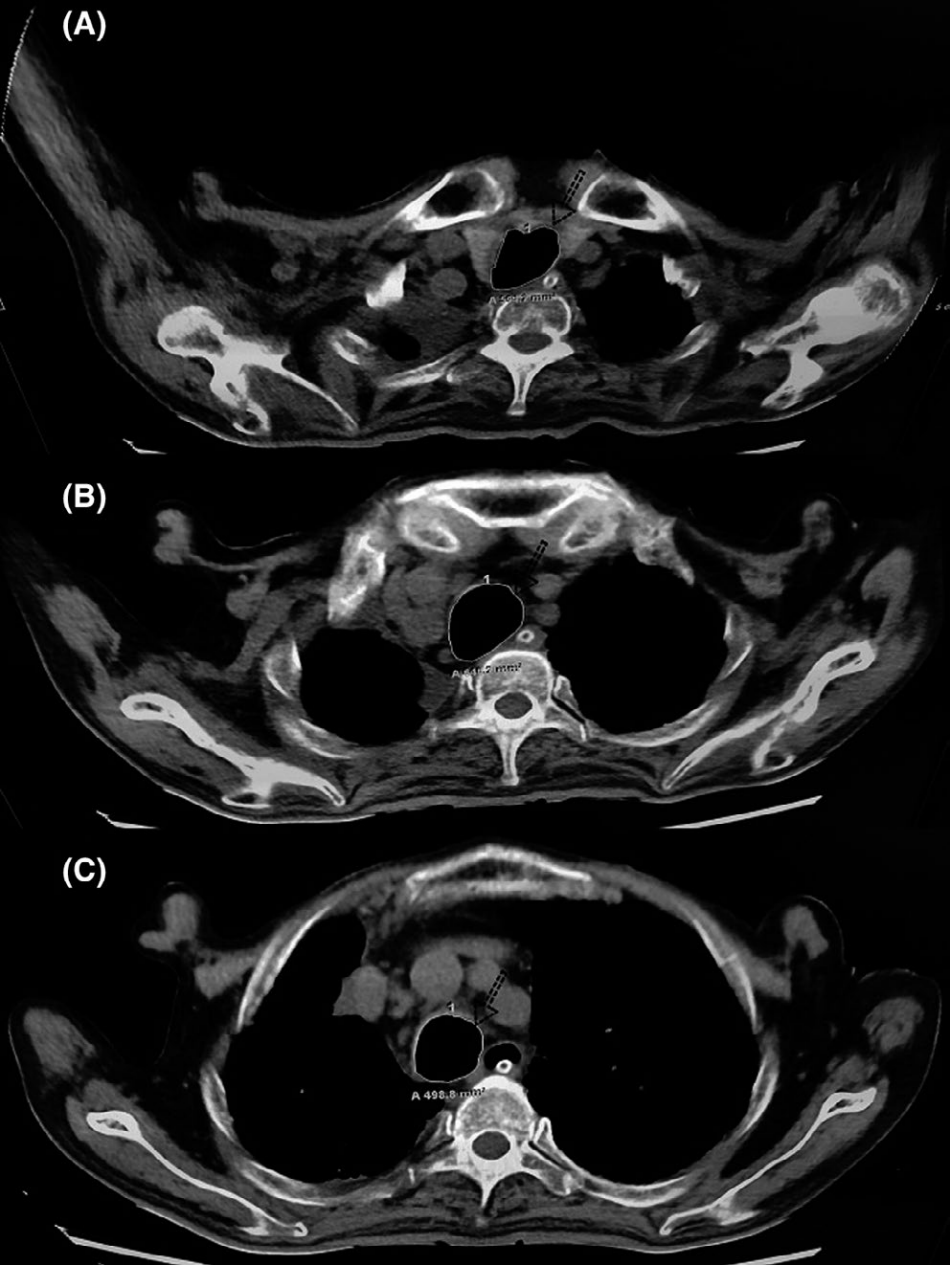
Computed tomography images used for measurement of tracheal cross‐sectional area at different upper vertebral levels. (A) T2 vertebra (584.2 mm^2^). (B) T3 vertebra (646.2 mm^2^). (C) T4 vertebra (498.8 mm^2^)
